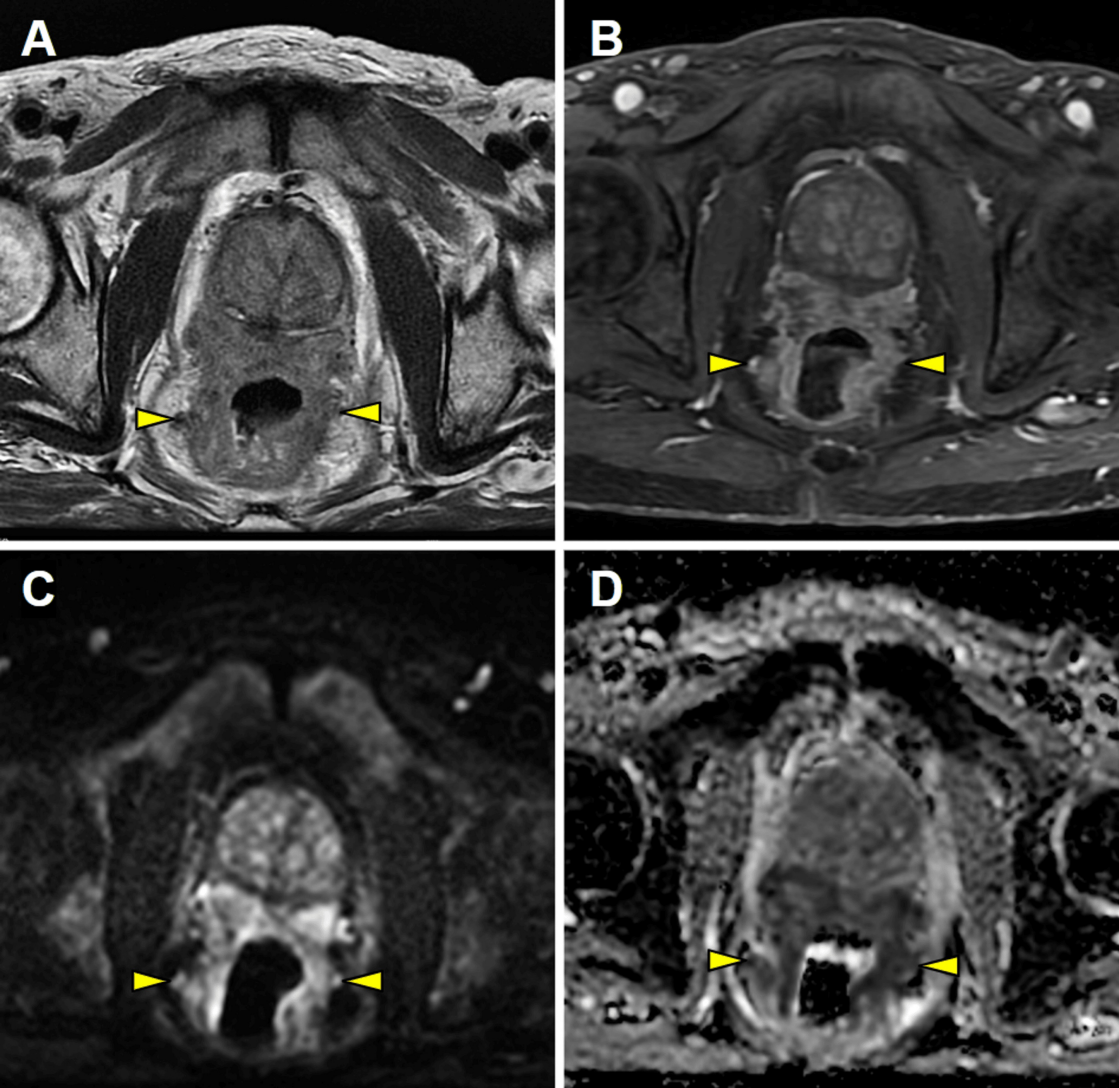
MRI study demonstrating a locally aggressive prostatic tumor MRI: magnetic resonance imaging Axial T2-weighted imaging (T2-WI) (A), gadolinium-enhanced fat-suppressed T1-weighted imaging (FS T1-WI) (B), diffusion-weighted imaging (DWI) (C), and apparent diffusion coefficient (ADC) map (D) reveal a large, locally aggressive prostatic tumor with invasion of the seminal vesicles, lymphovascular bundles, and rectal walls (arrowheads). The lesion demonstrates intermediate signal intensity on T2-WI, hypervascularity on gadolinium-enhanced FS T1-WI, and marked diffusion restriction, as evidenced by high signal intensity on DWI and corresponding low signal intensity on the ADC map
